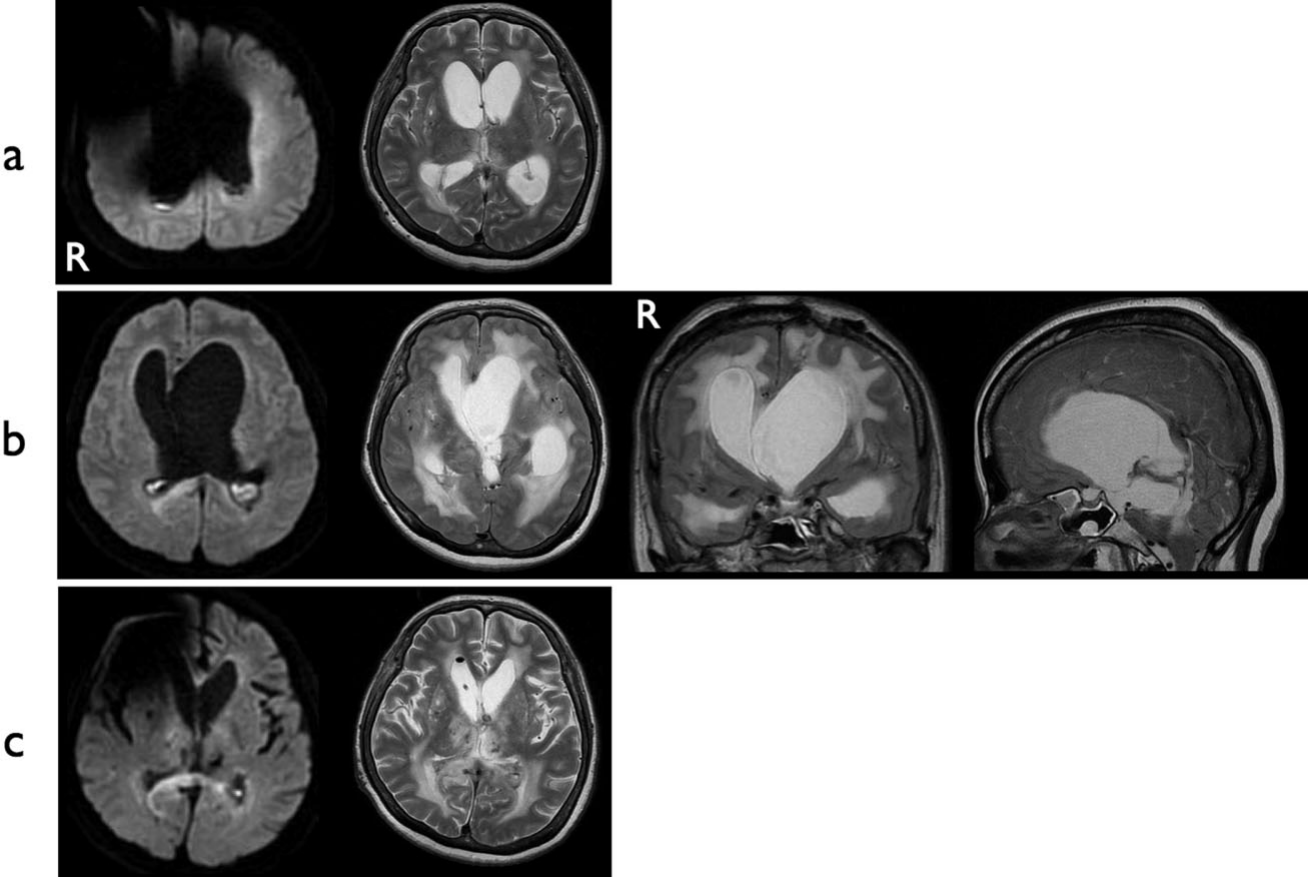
Case 2. (a) MRI on admission. DWI shows abnormal hyperintensities in the occipital horns of both lateral ventricles. Hydrocephalus is obvious. A metallic artifact is apparent around the right frontal region. (b) Preoperative MRI. Progression of asymmetrical hydrocephalus with findings of ventriculitis is evident. (c) Postoperative MRI. Both ventriculitis and hydrocephalus have resolved. DWI, diffusion-weighted imaging.
